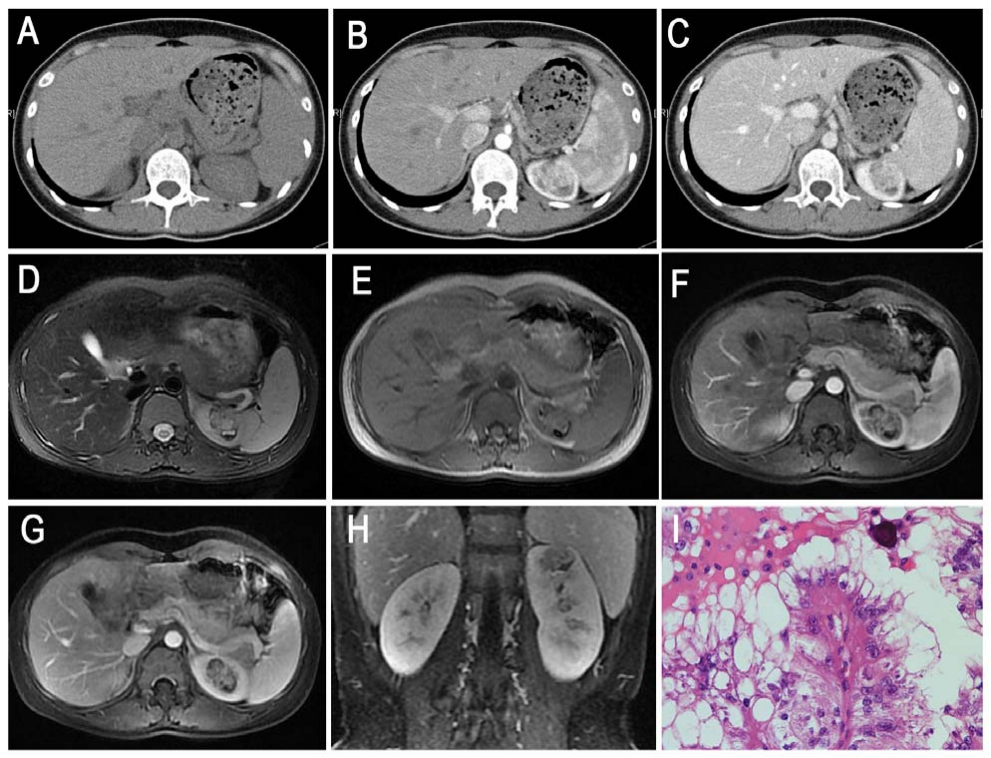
Translocation RCC in a 25-year-old woman (patient 8 in Tables 1, 2, 3) with an incidentally detected left renal mass on US. A, Axial unenhanced CT image showing an ill-defined, slightly high attenuation of the mass within the left kidney contour. B–C, Axial CMP and NP contrast-enhanced CT images demonstrating heterogeneous and prolonged enhancement of the mass in the left kidney. D–E, Axial T2WI and plain (non-contrast) T1WI showing an irregular ill-defined left renal mass with a heterogeneous, slightly high signal intensity, which seemed to break the capsule. Axial CMP (F) and NP (G) gradient-echo MR images showing heterogeneous moderate enhancement of the left renal mass. H, Coronal delay phase image showing delay enhancement of the mass. I, The tumor cells contain hyaline cytoplasm, eosinophilic cells with papillary structure and psammoma bodies (HE 10 & 40).
